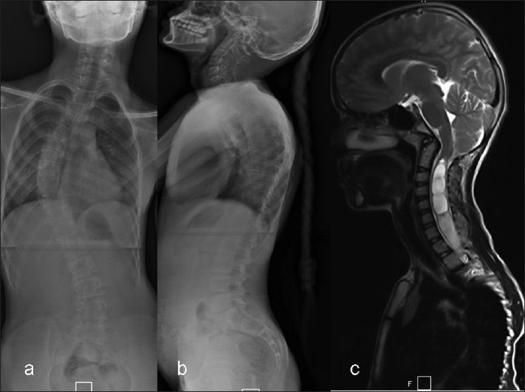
11 year old child with right thoracic presumed ‘idiopathic’ scoliosis. Clinically she had an asymmetrical abdominal reflex. (a, b) The radiographs showed a 53° right thoracic scoliosis with a thoracic kyphosis (T4-T12) of 38°. (c) The MRI showed a Arnold Chiari type-I malformation with a cervicothoracic syrinx. This required a foramen magnum decompression followed by posterior corrective instrumentation
